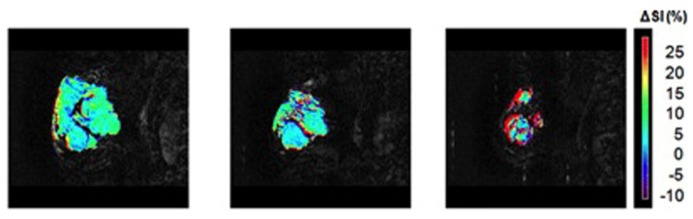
BOLD MRI for a patient with breast tumor exhibiting a partial response to therapy. Images show a signal enhancement maps (color) overlaid on T2-weighted anatomical images. Images have been acquired 1 week before start of neoadjuvant chemotherapy (left), after one cycle of chemotherapy showing small signal response (middle) and after four cycles of chemotherapy demonstrating a striking change in tumor characteristics in response to therapy (right). Adapted from Jiang and Weatherall (2013), reproduced with permission.
